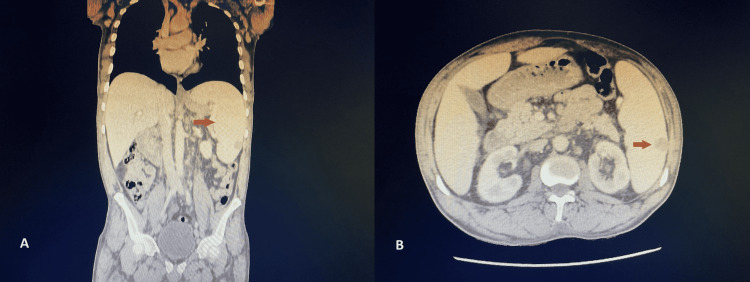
Abdominal computed tomography findings (A) Splenomegaly (red arrow) measuring 18 cm in diameter in coronal section. (B) Hypodense splenic nodule (red arrow) in cross-section.
